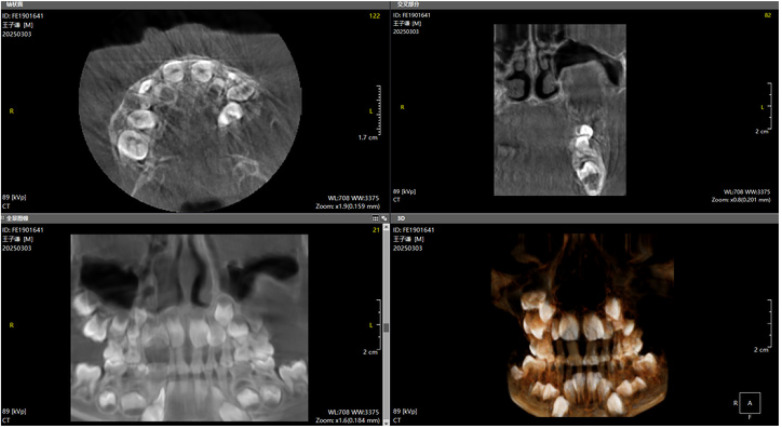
Postoperative CBCT image (2).
